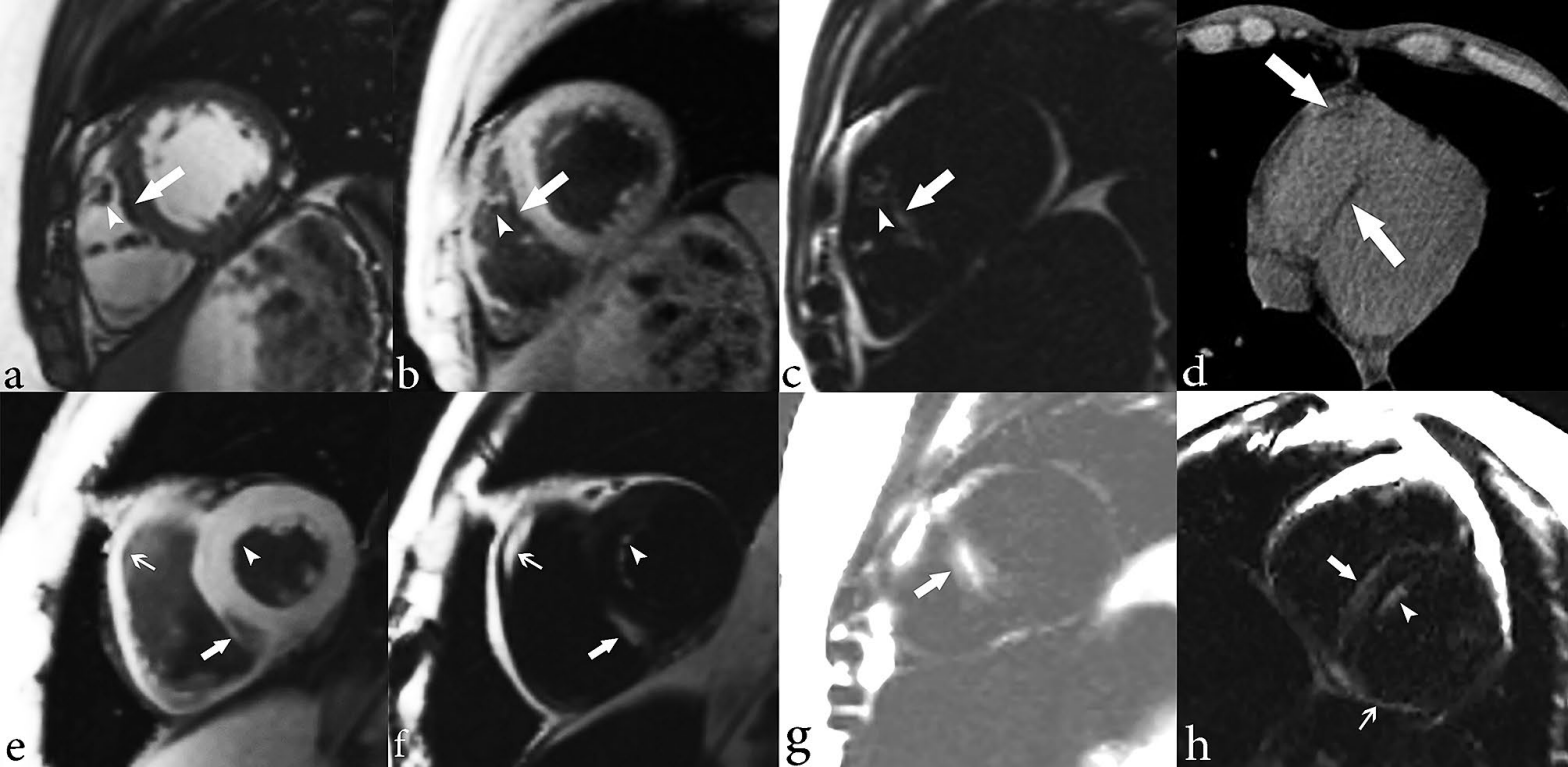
Cardiac MR images depicting intramyocardial fat in a 21-year-old female with Proteus syndrome. (a) Short axis cine image of a midventricular slice shows some areas of low signal intensity within the right ventricular (RV) trabeculae (arrowhead) and RV side of the interventricular septum (arrow) secondary to areas of chemical shift artifact caused by the presence of small areas of fat in the myocardium. Short-axis cardiac optimized multi-echo Dixon water (b) and fat (c) separation images of a mid-ventricular slice. (b) Short axis water image of the mid heart shows areas of intramyocardial fat as low signal intensity, for example within the RV trabeculae (arrowhead) and along the RV side of the interventricular septum (arrow). The fat image (c) shows areas of high signal intensity compatible with fat within the RV trabeculae (arrowhead) and RV endocardial side of the interventricular septum (arrow). (d) CT image of the chest confirms areas of fat within the RV side of the interventricular septum and right ventricular free wall of the heart (arrows). Patterns of intramyocardial fat are depicted on (e–g). Short axis water image (e) of a mid LV slice shows areas of midwall (thick arrow) and subendocardial (arrowhead) fat in the left ventricle as areas of low signal intensity. There is also fat involving the RV free wall (thin arrow). A corresponding fat image (f) shows the midwall (thick arrow), subendocardial (arrowhead), and RV wall fat (thin arrow) as areas of high signal intensity. Note that the subendocardial fat which is difficult to appreciate on the water image because of its similar signal intensity to the blood pool is very obvious on the fat image. (g) Short axis fat image of the mid LV shows a transmural area of high signal intensity corresponding to transmural fat within the septal wall (arrow). (h) Short axis fat image of a mid LV slice shows areas of fat as high signal intensity in several distributions: epicardial in the inferior wall (thin arrow), endocardial on the RV side of the interventricular septum (thick arrow) and endocardial on the LV side of septal wall (arrowhead).
